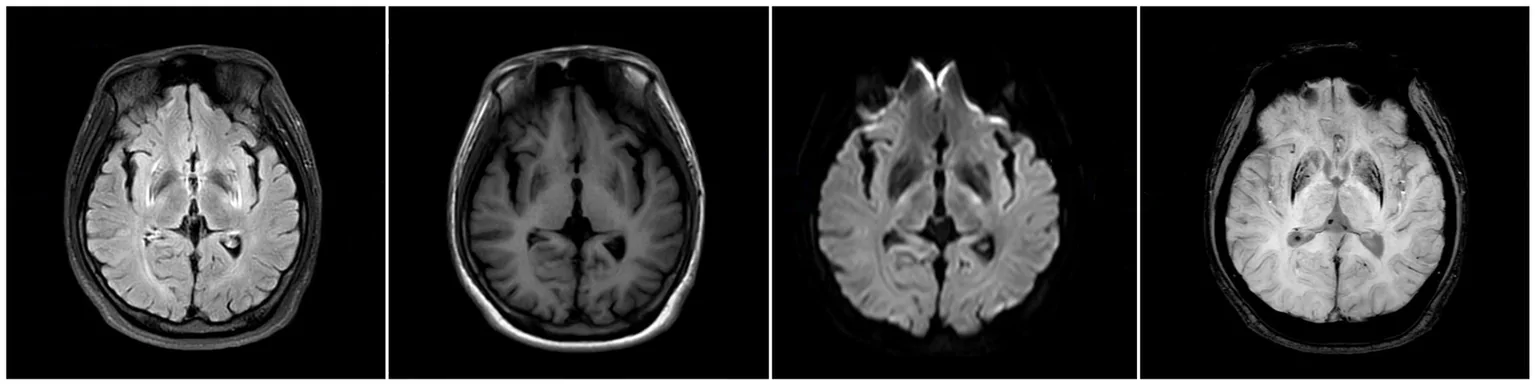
Brain MRI revealed abnormal signals in the bilateral putamen.
